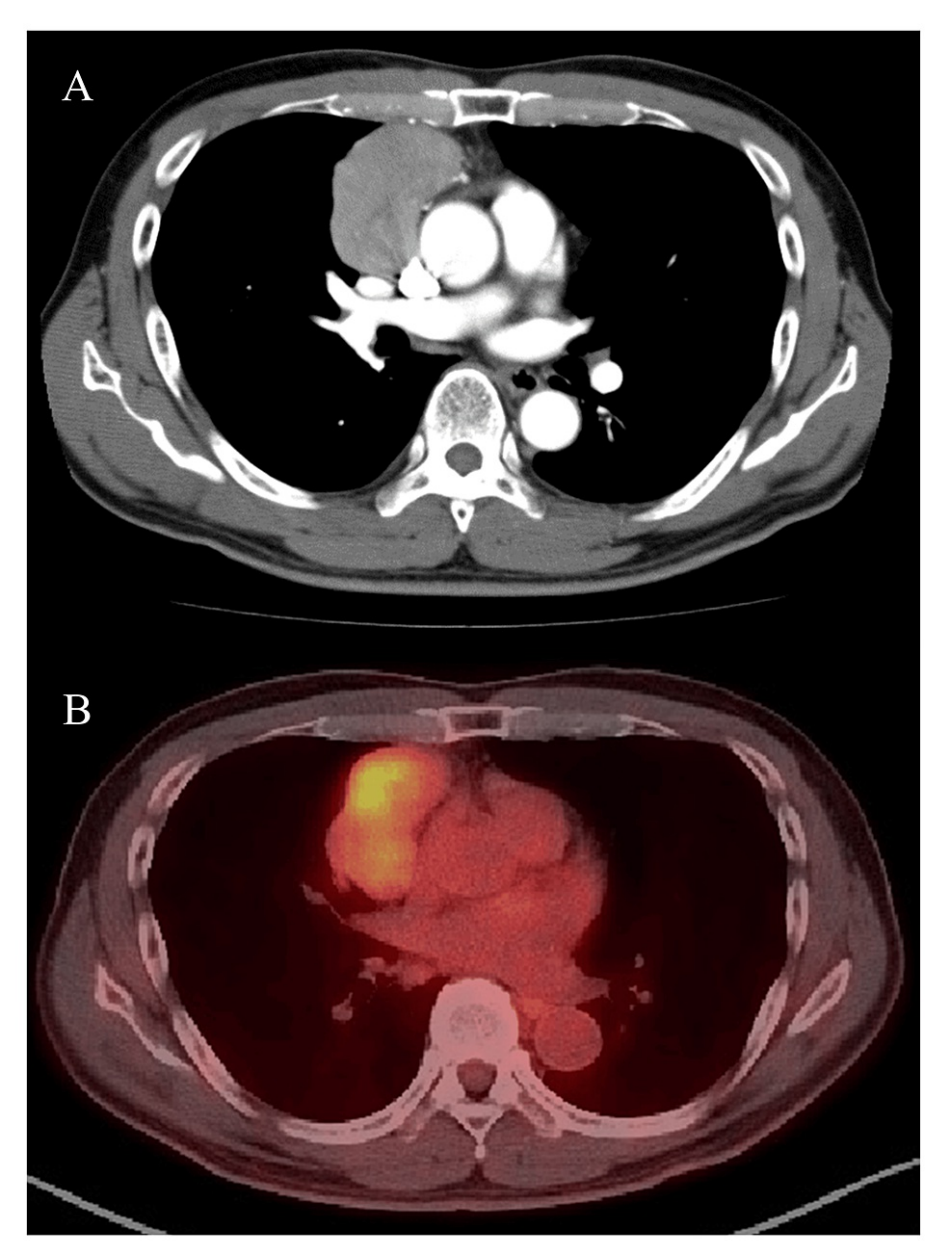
Radiologic findings at diagnosis A. Contrast-enhanced computed tomography shows a poorly contrasted anterior mediastinal tumor. B. ^18^F-fluorodeoxyglucose (FDG) positron emission tomography showed an FDG-avid anterior mediastinal tumor.
